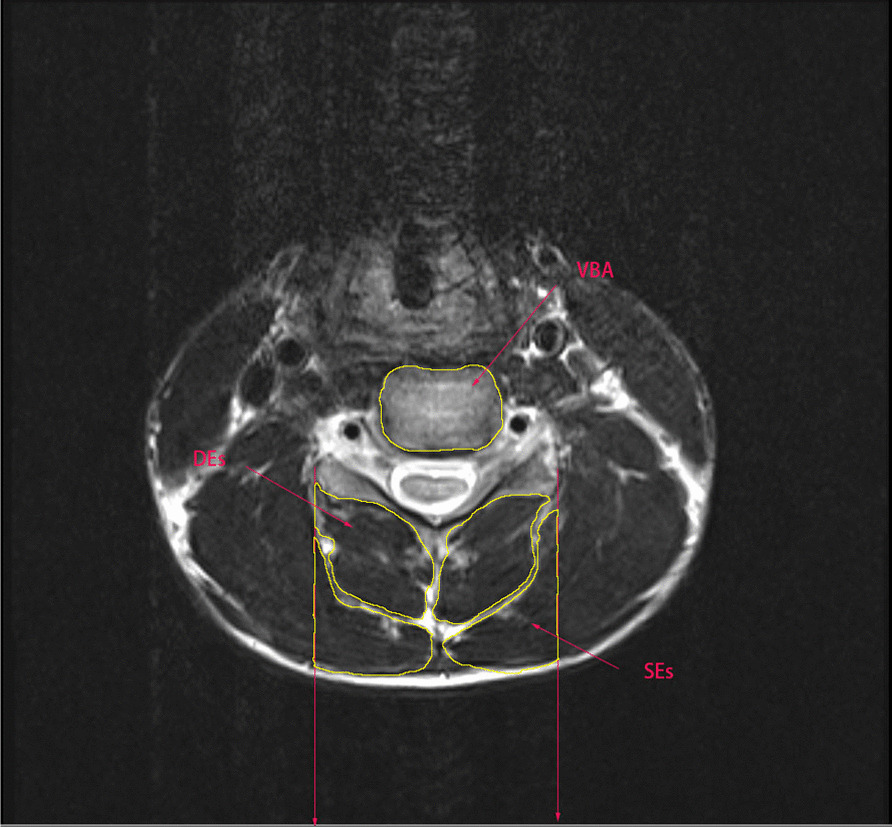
Demonstration of measuring regions of intrest on T2-weighted axial MR
